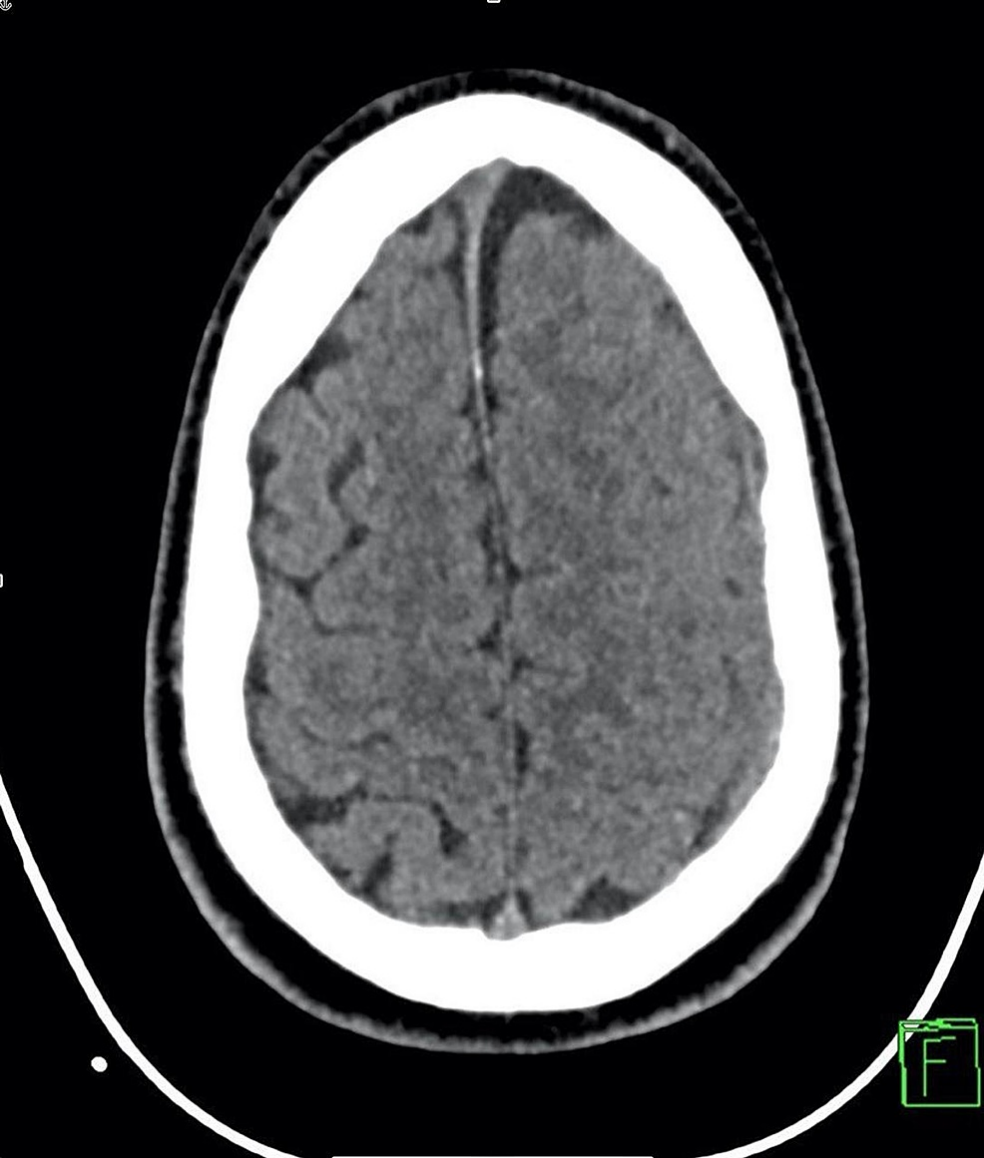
CT brain axial view: minimal left chronic residual subdural hematoma with no significant mass effect or midline shift.
